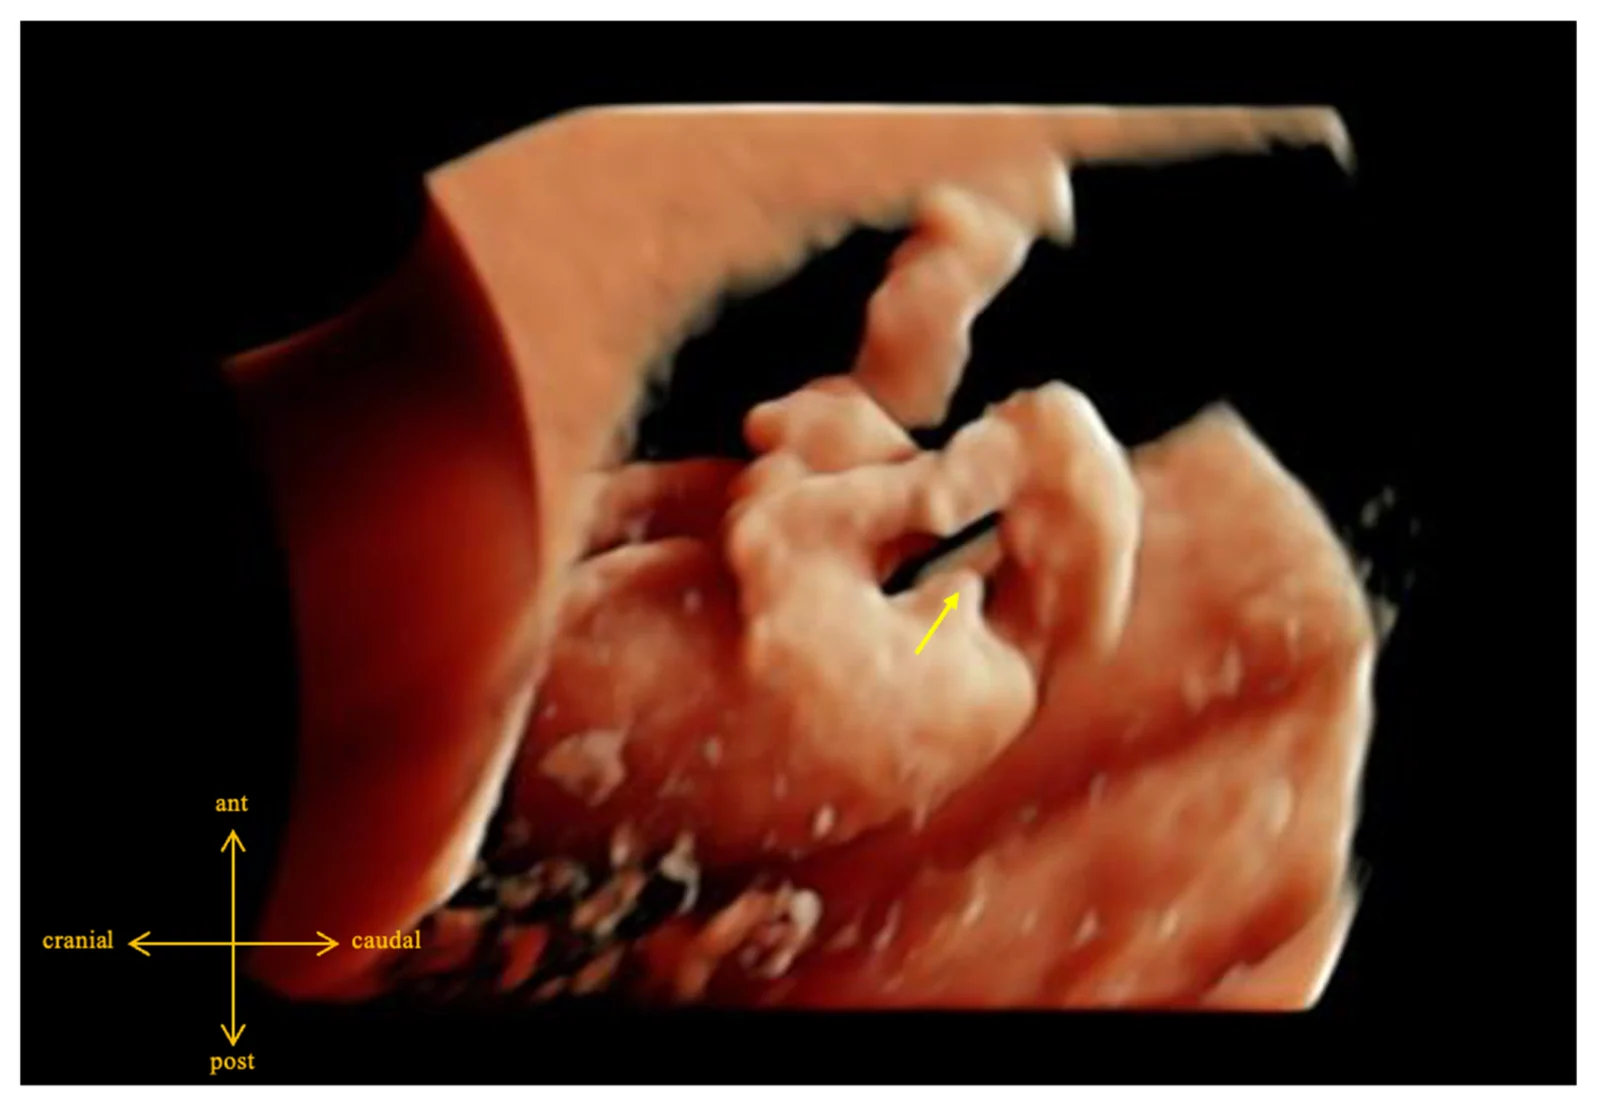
Ultrasonographic aspect of 8-week male individual. Note the morphology of the genital tubercle, along with its cranial direction, as it is seen from a sagittal plane (yellow arrow).
